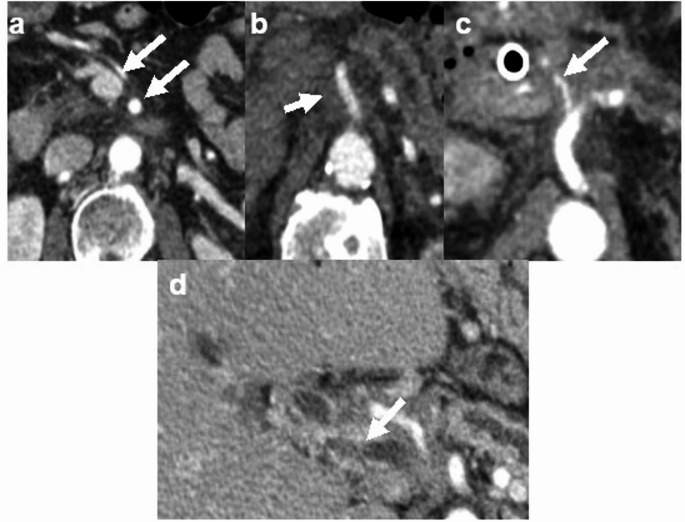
Axial contrast enhanced CT images depict grading of vascular involvement. a No vascular involvement of superior mesenteric vessels, grade 0 (arrows). b Encasement of the superior mesenteric artery without stenosis, grade 1 (arrow). c Encasement with stenosis of the common hepatic artery, grade 2 (arrow). d Encasement and occlusion of the main portal vein, grade 3 (arrow)
